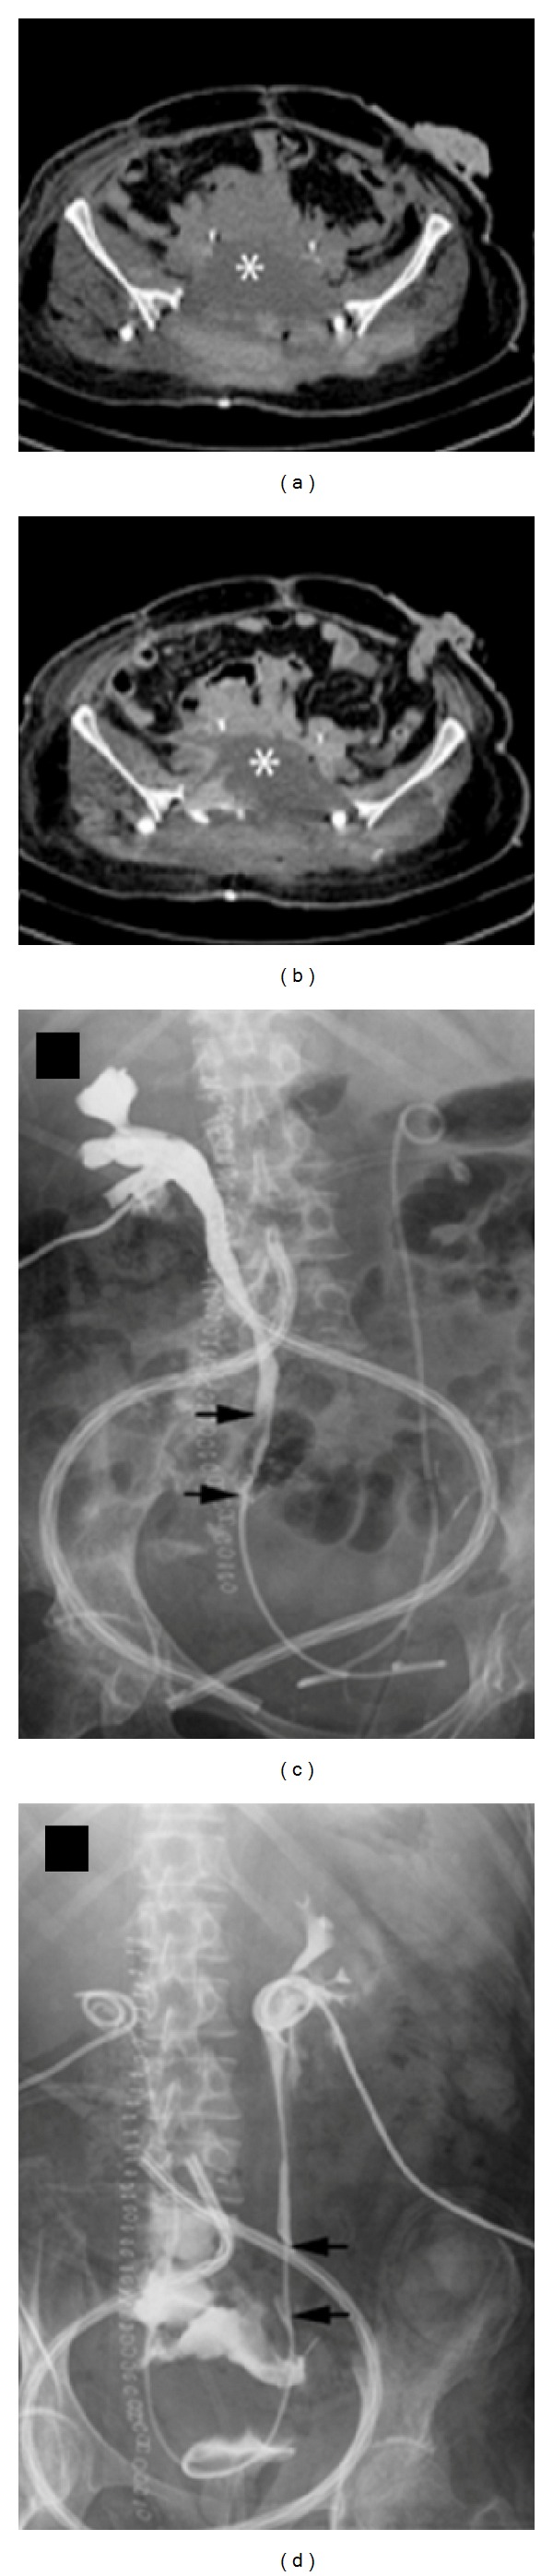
Noncontrast-enhanced (a) and contrast-enhanced (b) CT scans of the pelvis after radical sacrectomy. A large fluid collection (white star) surrounds the ureters where two double-J stents were positioned. Pyelography through the bilateral nephrostomies showing the transection of both ureters (black arrows) and contrast media leakage ((c) and (d)).
